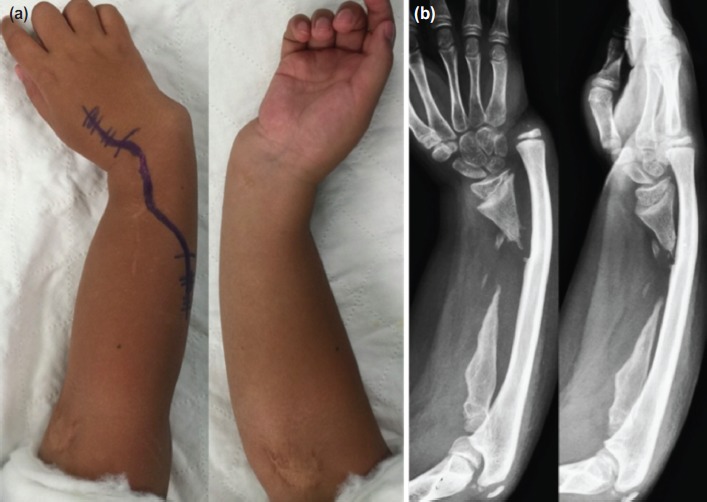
(a) Pre-operative photograph of the child showing radial clubhand deformity. (b) Radiographs showing loss of diaphysis of the right radius, intact epiphysis and hypoplastic radial head.
